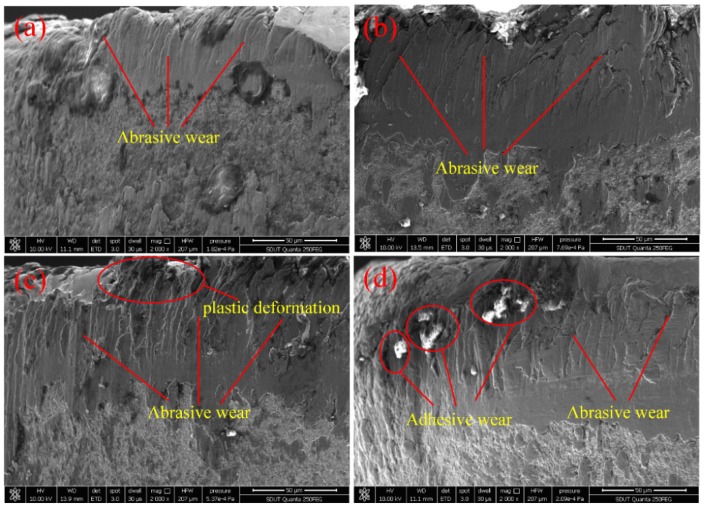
Tool morphology under ultrasonic atomization assisted machining at four cutting speeds: (a) 30 m/min, (b) 40 m/min, (c) 50 m/min, and (d) 60 m/min.
